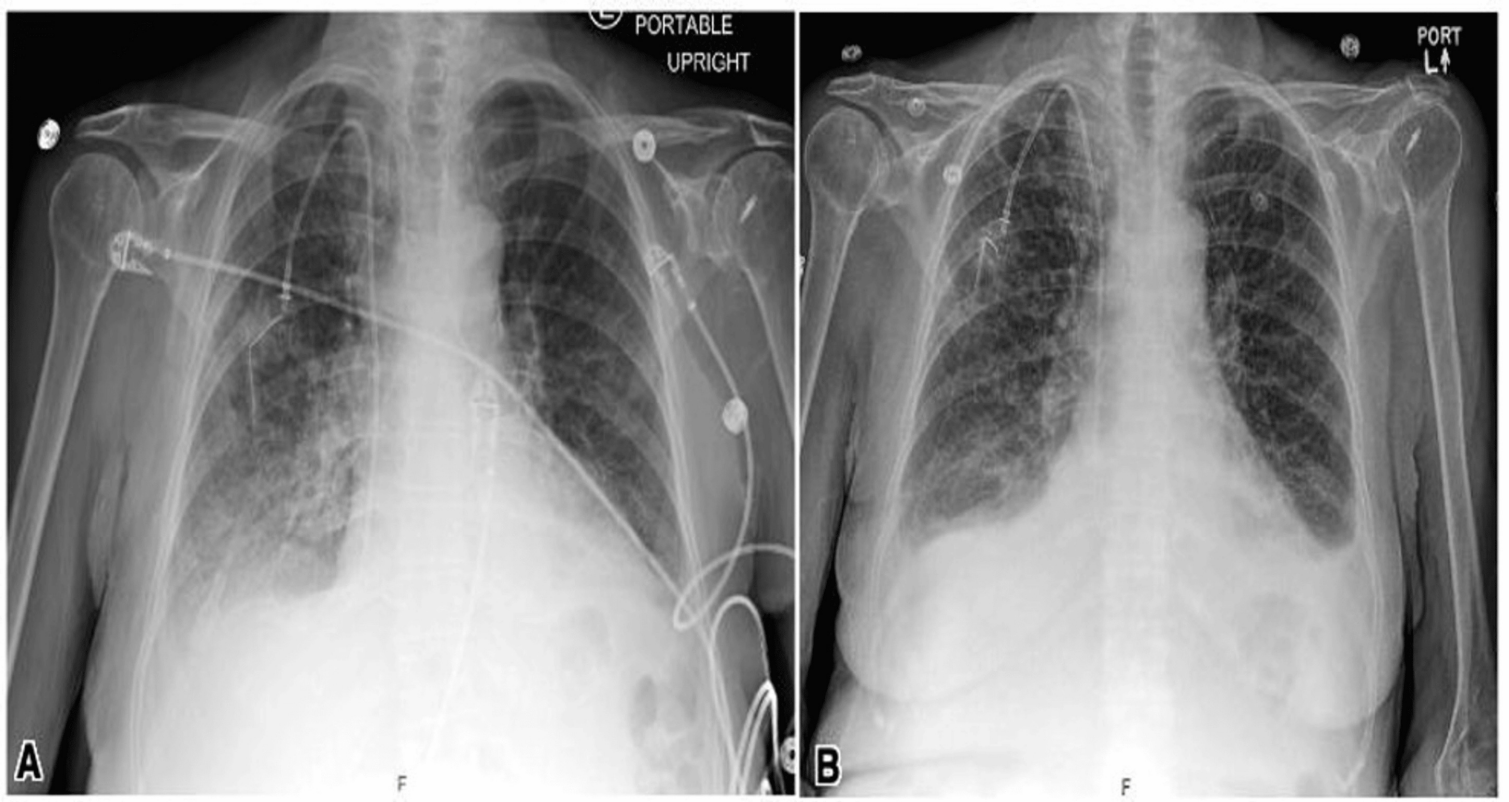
Chest X-ray on presentation showing pulmonary edema (A). Chest X-ray on hospital day 5 showed venous congestion and mild-to-moderate edema with bilateral small effusions (B).
